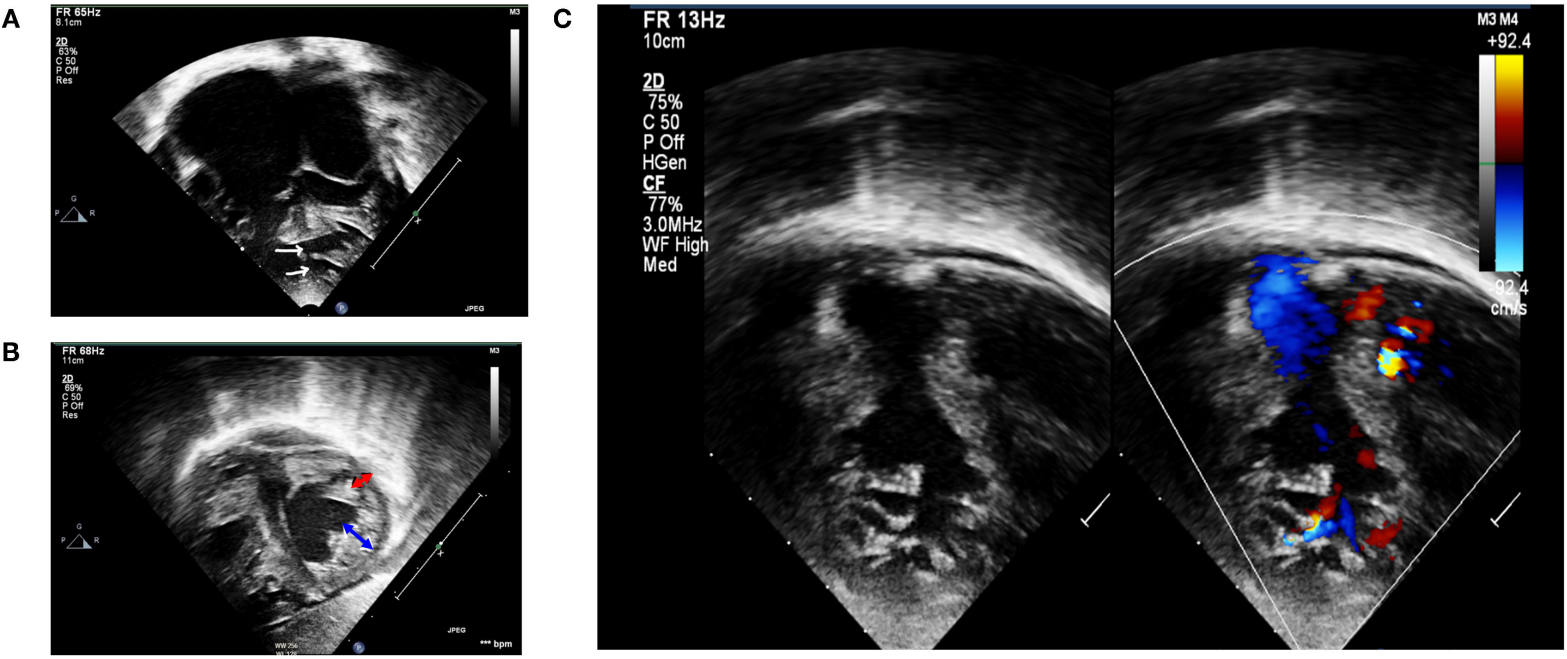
Transthoracic echocardiography. (A) Apical four-chamber view showing biventricular hypertrophied heart with multiple mid and apical-muscular VSD (white arrows); markedly dilated atria, especially the right atrium. (B) Subcostal short-axis view in end-systole revealing non-compaction cardiomyopathy involving both ventricles. Non-compaction area is depicted by blue arrow, whereas compaction area is depicted by red arrow. (C) Left panel: 2D echocardiography demonstrating RV non-compaction with multiple and prominent trabeculation with deep recesses; right panel: a color Doppler echocardiography demonstrated direct communication between RV cavity into deep intertrabecular recesses.
